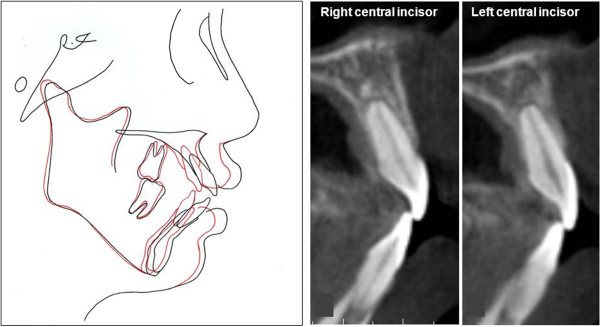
Superimposition and sagittal image of upper central incisors after debonding using CBCT of case 1. Both of them were well maintained within the alveolar housing.
